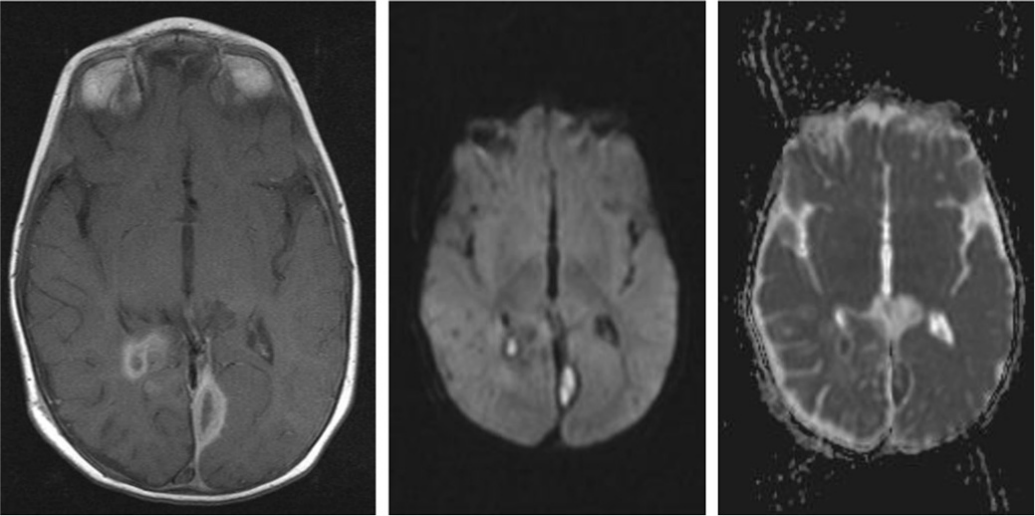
Magnetic Resonance Imaging 6 weeks after admission.
